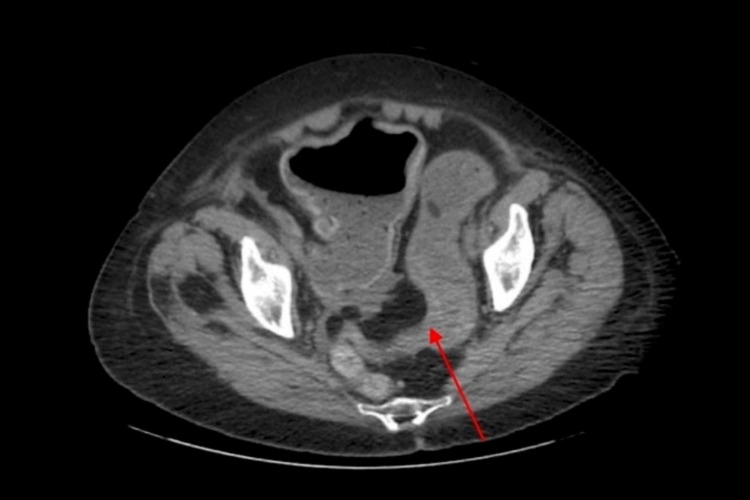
CT abdomen and pelvis revealed diffuse distention of the colon leading up to an abrupt bird beak transition to a collapsed colonic segment at the mid to distal sigmoid (red arrow)
